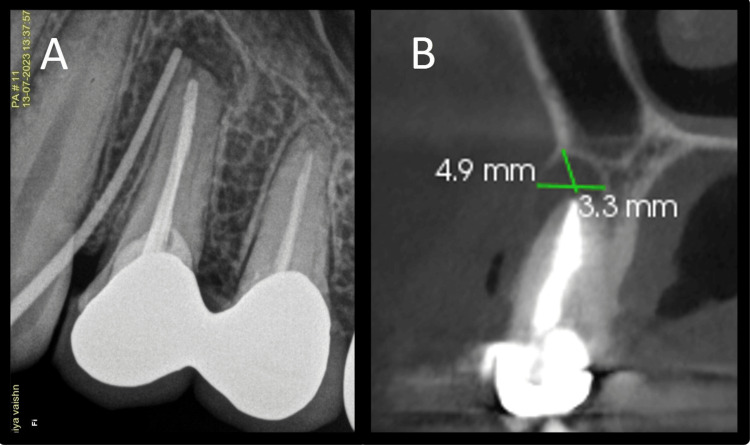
Sinus tract tracing and cone beam computed tomography image A: Sinus tract tracing, B: Cone beam computed tomography coronal view
